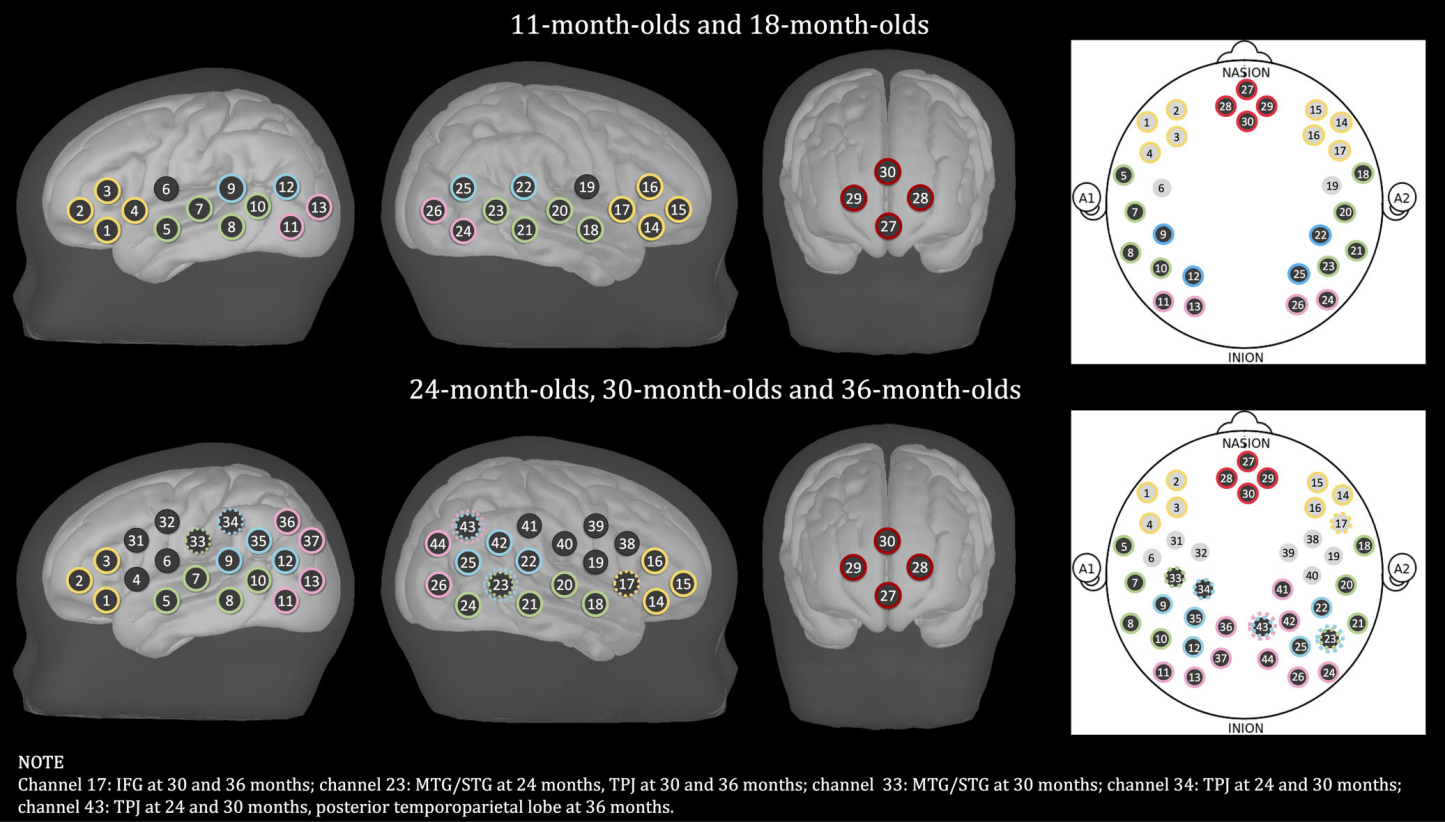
Representation of the channels on a MRI template both for the 30‐channel and the 44‐channel configuration. ROIs are colour‐coded: Red represents mPFC; yellow represents IFG; green represents MTG/STG; blue represents TPJ; pink represents posterior temporoparietal lobe. Dotted circles mark channels belong to different ROIs at different ages
